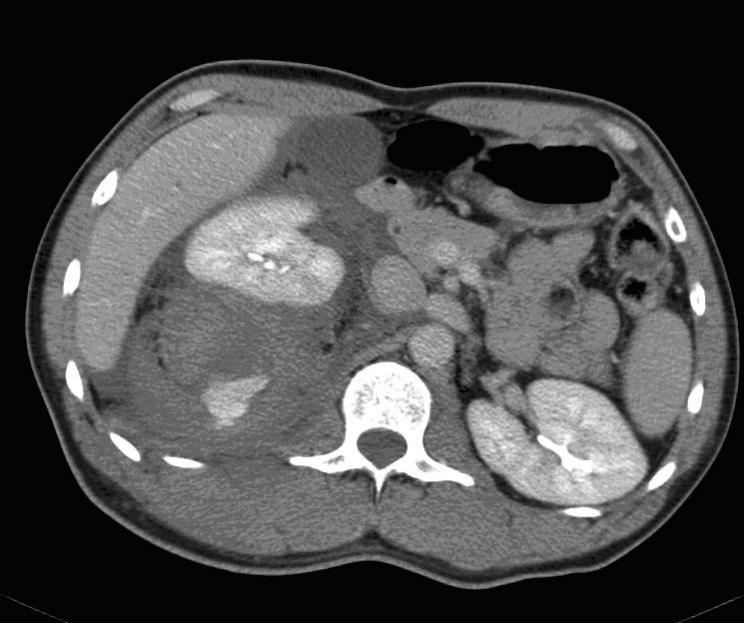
Computed tomography with intravenous contrast demonstrating large hematoma around the right kidney with contrast extravasation.
